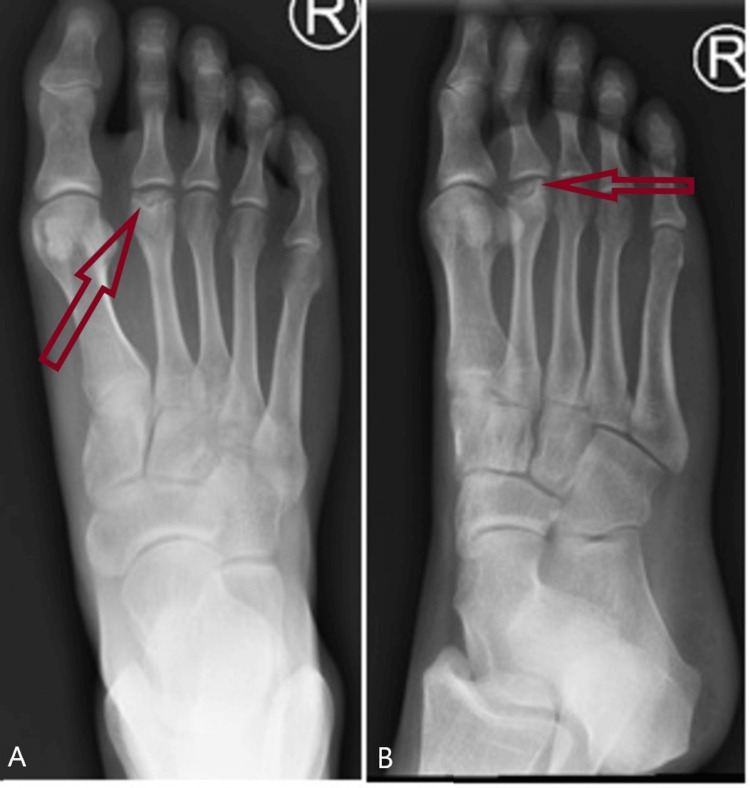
X-rays show flattening of the right second metatarsal head. A: AP X-ray showing flattening of the right second metatarsal head B: Oblique X-ray showing flattening of the right second metatarsal head
